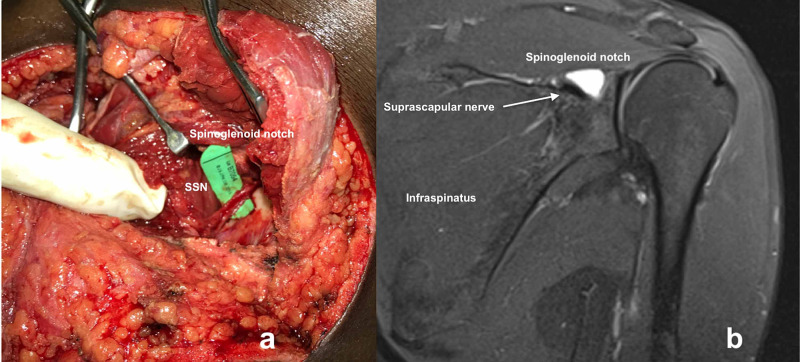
(a) Intraoperative pictures showing the spinoglenoid notch and decompressed suprascapular nerve (SSN). (b) MRI images correlating the intraoperative pictures showing the spinoglenoid notch, cysts and decompressed suprascapular nerve. The nerve runs inferolateral in the spinoglenoid notch and curves around the lateral margin of the scapular spine to enter the infraspinatus fossa.
